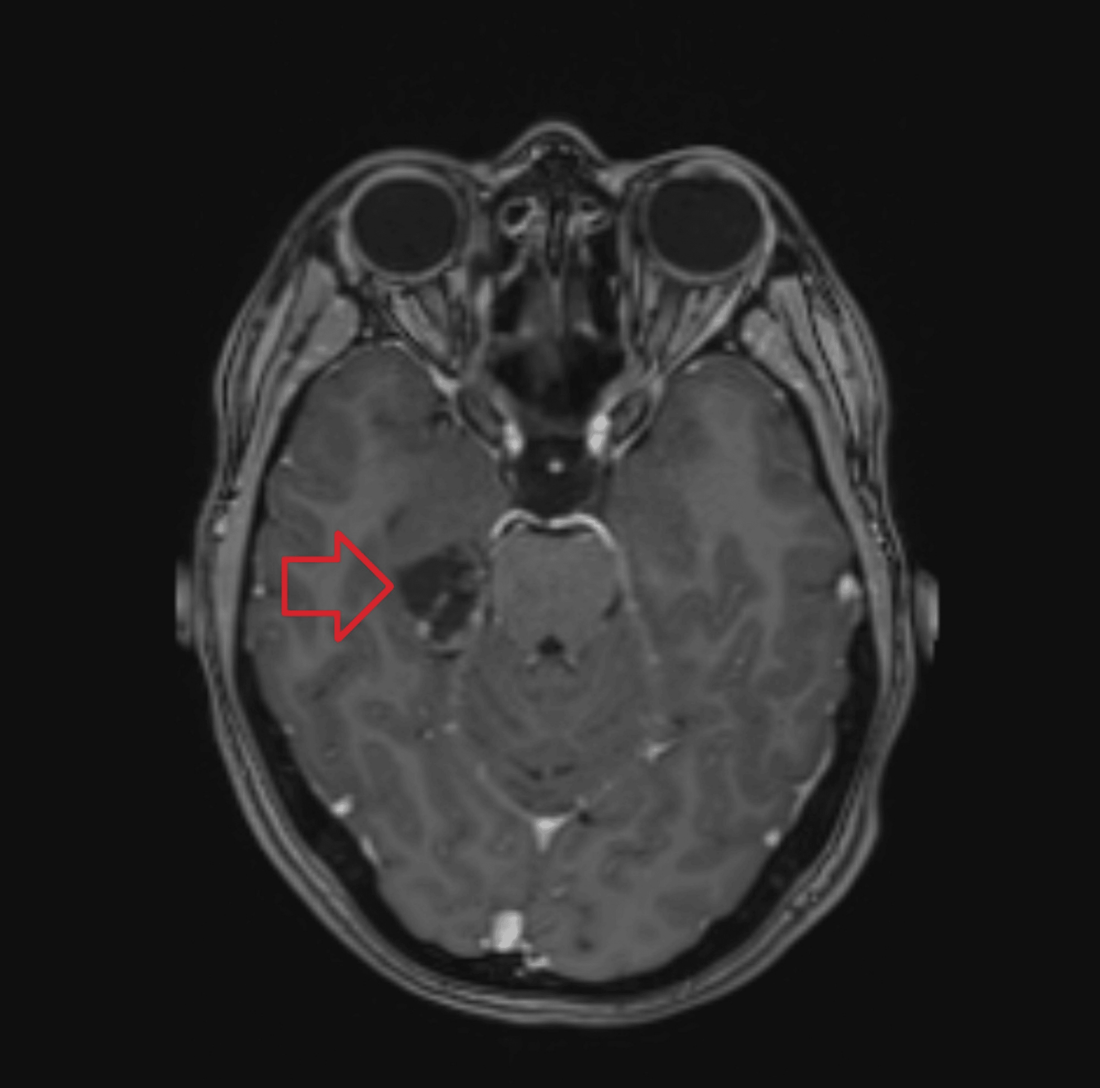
MRI of the right temporal lobe showing a lesion posterior to the hippocampus suggestive of pilocytic astrocytoma
